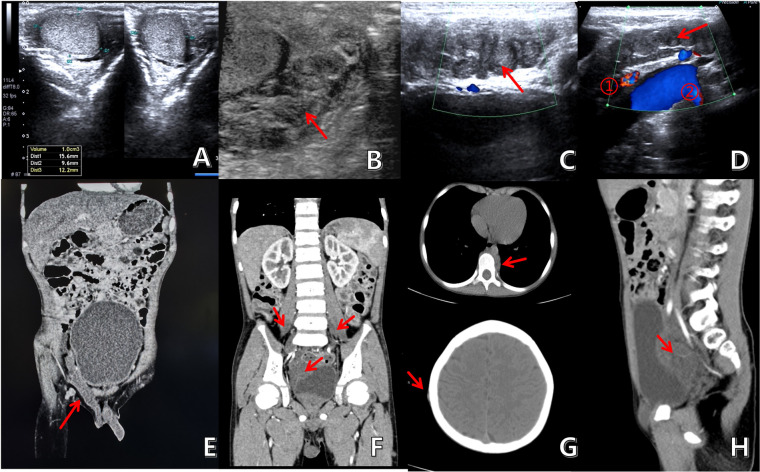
Ultrasonographic and CT findings of an extratesticular inguinoscrotal lesion with deep pelvic and retroperitoneal extension. (A) Scrotal ultrasonography showing the right testis with preserved contour and homogeneous echotexture, without focal intratesticular abnormality; the measured dimensions on the representative image were approximately 15.6 × 9.6 × 12.2 mm. (B) Irregular extratesticular lesion located posterior and superior to the right testis. (C) Beaded/nodular extratesticular lesions extending along the right inguinal course. (D) Color Doppler ultrasonography showing the inguinal lesion in close relationship to the iliac vessels, with ① indicating the iliac artery and ② indicating the iliac vein. (E) Coronal CT showing right inguinoscrotal extension contiguous with a large pelvic mass. (F) Coronal contrast-enhanced CT showing multiple irregular retroperitoneal and pelvic masses, including lesions adjacent to the iliac vessels. (G) Additional multifocal lesions on CT: the upper image shows a thoracic paravertebral lesion, and the lower image shows a right parietal scalp/subcutaneous lesion. (H) Sagittal CT showing a large pelvic mass posterior to the bladder with substantial craniocaudal extent. Red arrows indicate representative lesions or anatomic relationships in each panel.
